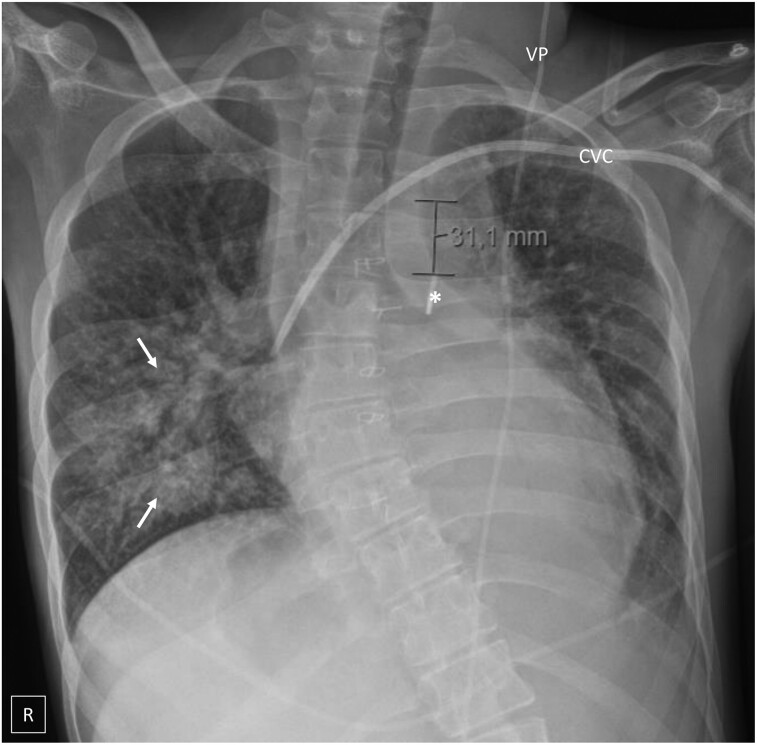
Chest X-ray on Day 3 showing consolidation of the right lower lobe (arrows), generalized alveolar shadowing and upper lobe venous diversion, as well as a central venous catheter (CVC) and ventriculoperitoneal shunt (VP). The X-ray demonstrates intra-aortic balloon pump placement with the radiopaque marker (asterisk) roughly 3 cm distal to the aortic knob, deliberately lower than standard placement due to aortic arch hypoplasia and previous surgical procedures.
